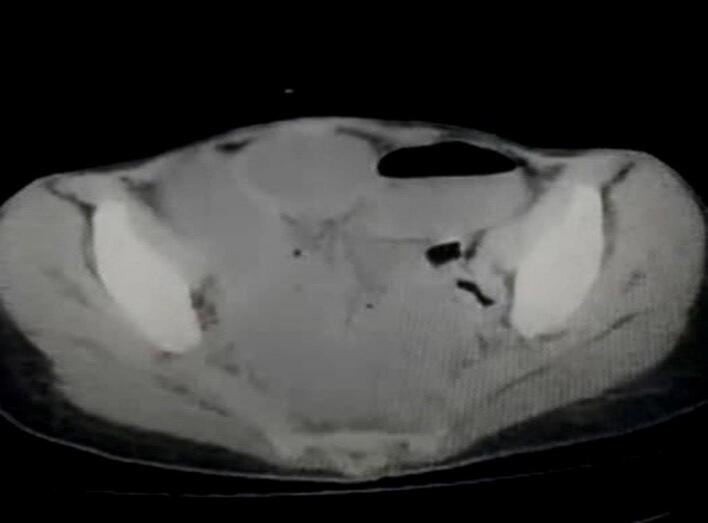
Axial CT scan demonstrates a radiopaque foreign body within the intestinal lumen, accompanied by mesenteric lymphadenopathy, ascites, and perilesional inflammatory stranding.
